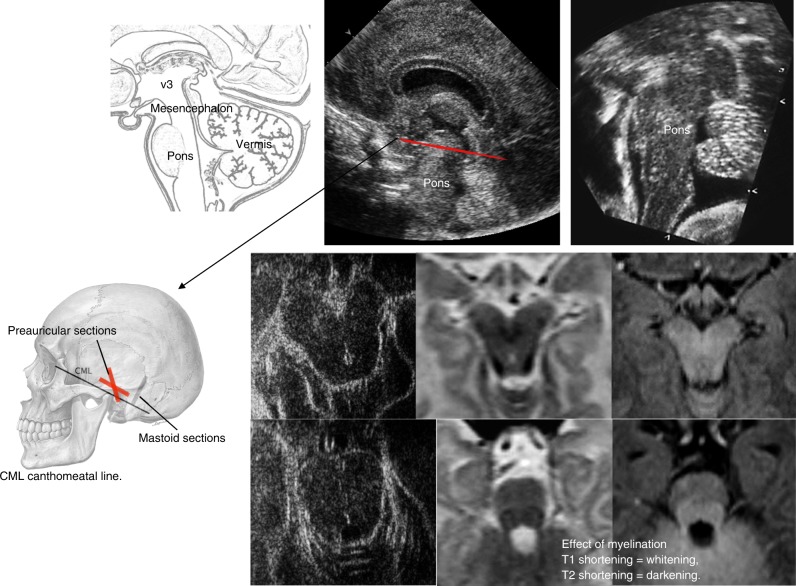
Technique and reporting: temporo-squamosal section. Top: sagittal section through brainstem and cerebellar vermis, compared with a sagittal scan of an infant of 27 weeks gestation, taken though the anterior fontanel with an 8.5 MHz scanhead; far right: sagittal 7.5 MHz ultrasound section of the area, taken through the posterior fontanel of an infant with cleidocranial dysplasia. Bottom: temporo-squamosal sections (parallel to the cantho-meatal line, indicated in red on the top scan): the echopoor mesencephalon looks like a butterfly; the cerebral peduncles and tectal lamina are surrounded by hyperechoic cisterns and parts of the tentorium; bright reflections in the posterior part of the brainstem coincide with the walls of the aqueduct; in the basal cisterns, the arteries of the circle of Willis show as short, pulsating lines; term MRI sections for comparison.
